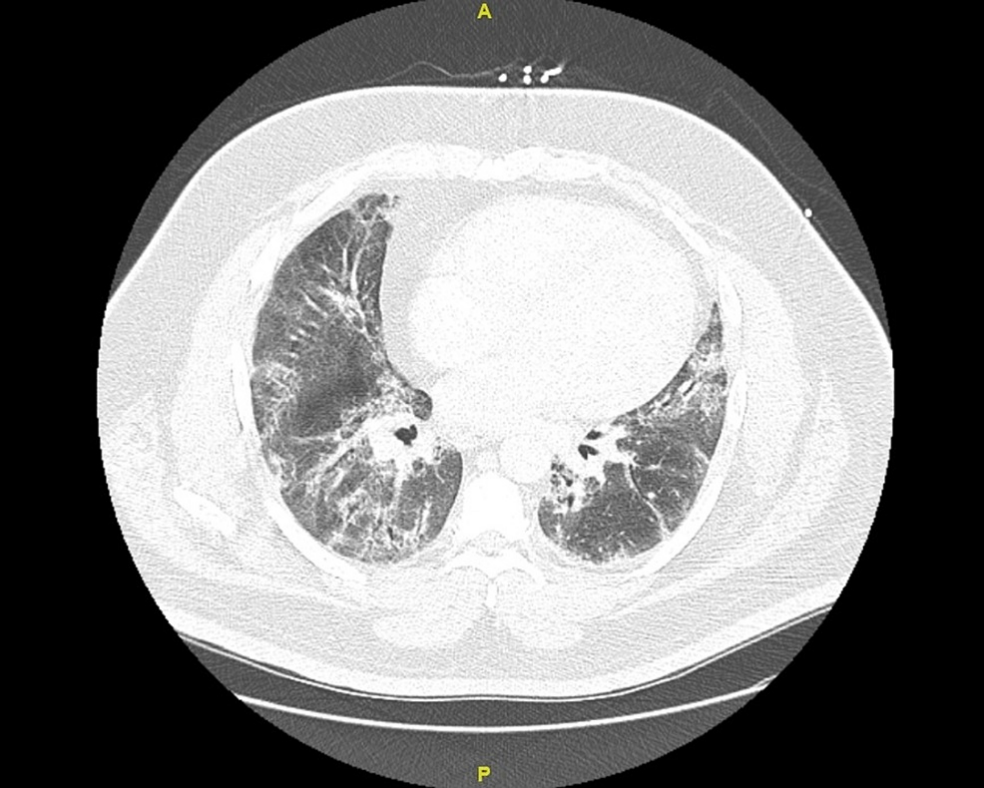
CT scan of the chest: Development of pulmonary fibrosis and bronchiectasis with scarring present at the periphery of the lungs
